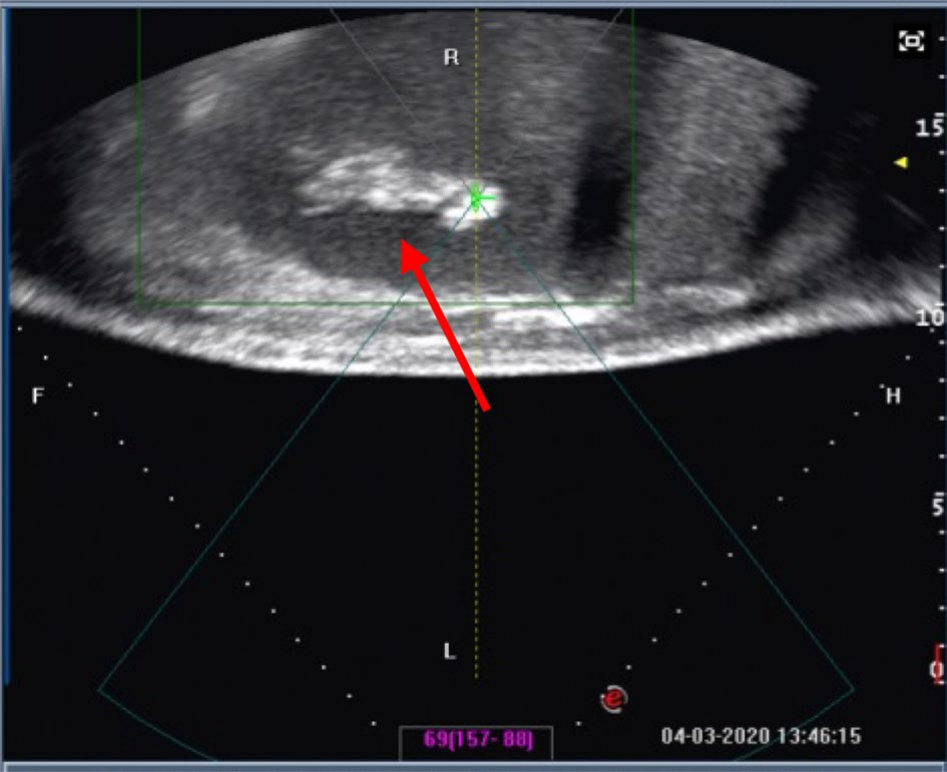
Real-time grayscale sonographic changes during high-intensity focused ultrasound treatment. The real-time sonography showed a hyperechoic area in the HIFU treated region of the spleen (red arrow).
